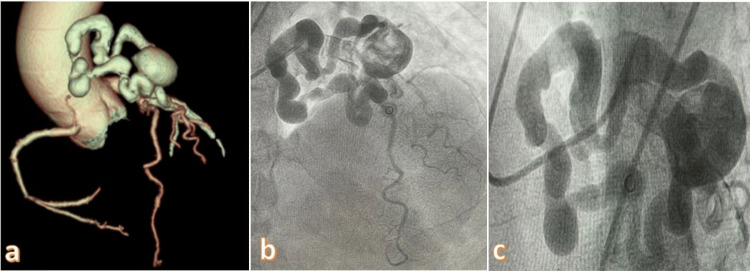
a) Aneurysm and collaterals caused by the coronary-pulmonary artery fistula on coronary CTA 3D imaging. b) Angiogram image of the coronary pulmonary artery fistula. c) Aneurysm and collaterals of the fistula. CTA: Computed tomography angiography
